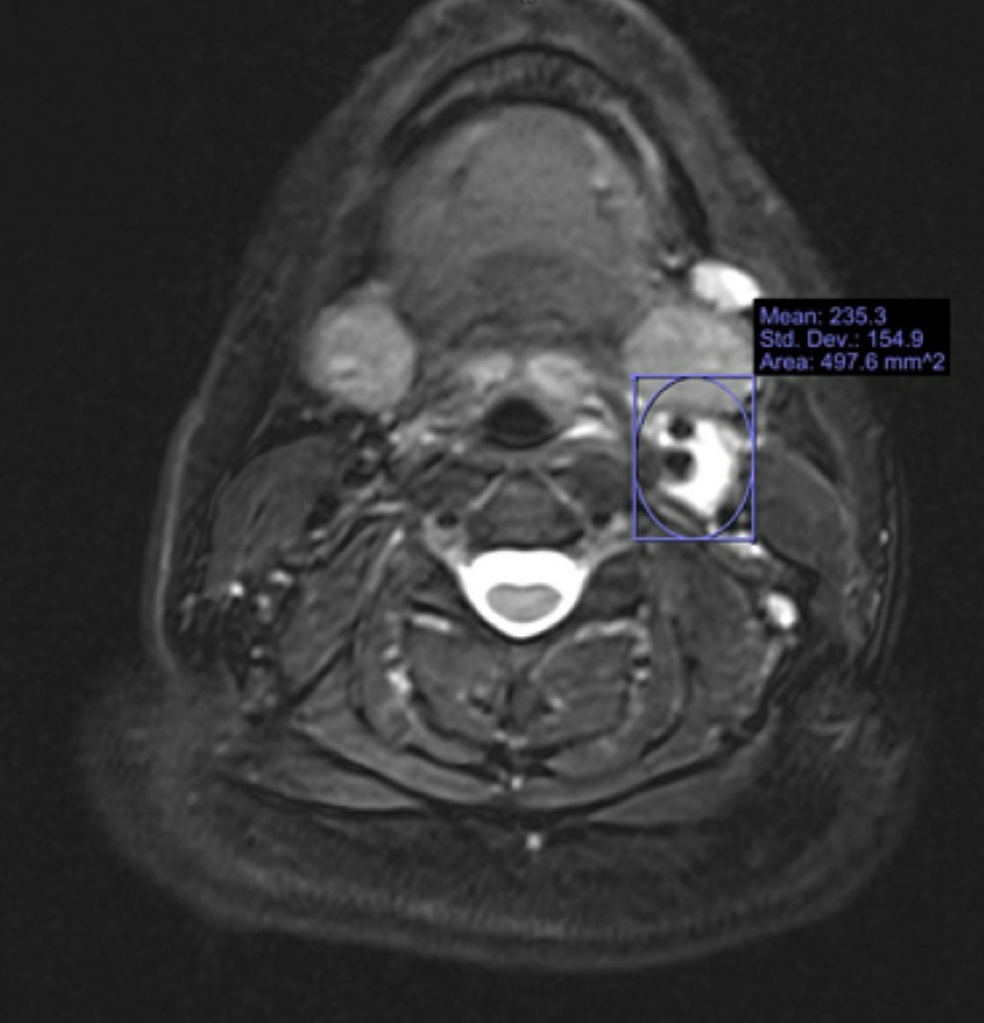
MRI soft tissue neck. Optical axial diffusion-weighted magnetic resonance image reveals hyperintensity and postcontrast enhancement surrounding the distal left common carotid, at the left carotid bifurcation, and proximal left internal carotid artery. Image obtained from Upstate Medical Department of Radiology.
